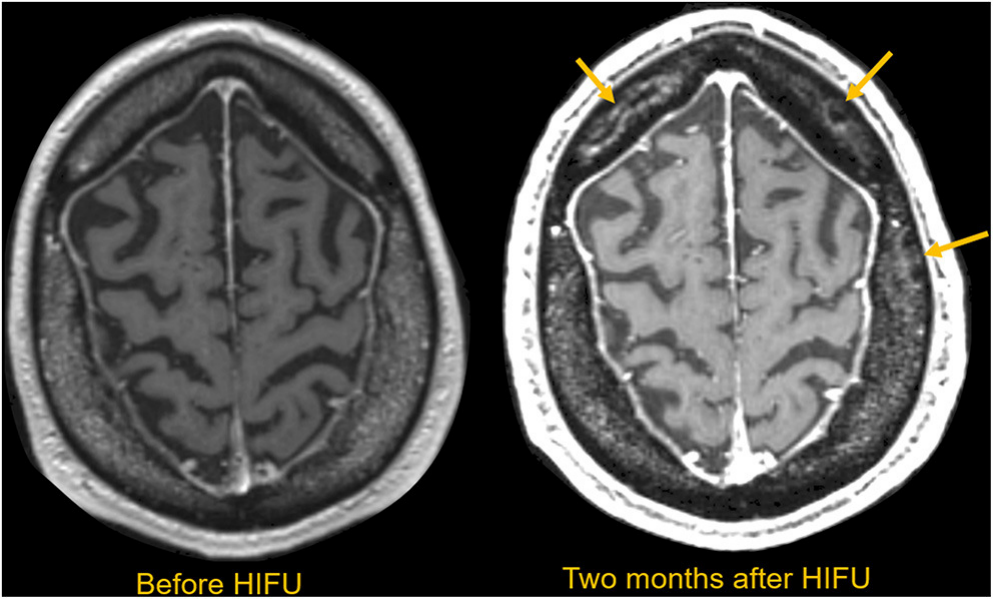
MRI scan obtained before and 2 months after HIFU in a patient requiring extended sonications to make a durable thalamic lesion. Multiple serpiginous marrow lesions compatible with infarctions are subsequently seen in the marrow space, as indicated with yellow arrows. These lesions are asymptomatic, but notable.
